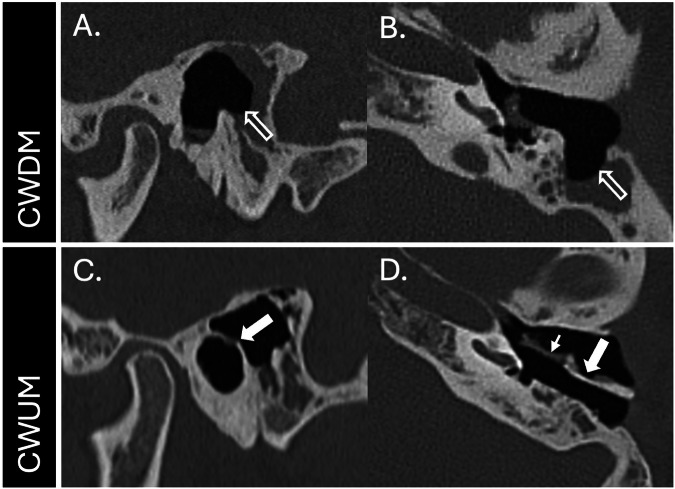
Mastoidectomy types. Sagittal (A) and axial (B) reconstructions from an unenhanced CT through the left temporal bone of a patient who has previously undergone CWDM. There is deficiency of the posterior EAC wall and the formation of a cavity (outlined arrows), which needs to be cleaned under otoscopic guidance. Sagittal (C) and axial (D) CT reconstructions in another patient who has previously undergone a CWUM with an intact posterior EAC wall (thick arrows). A defect in the superomedial EAC wall and tympanic membrane has been repaired with tragal cartilage (small arrow)
